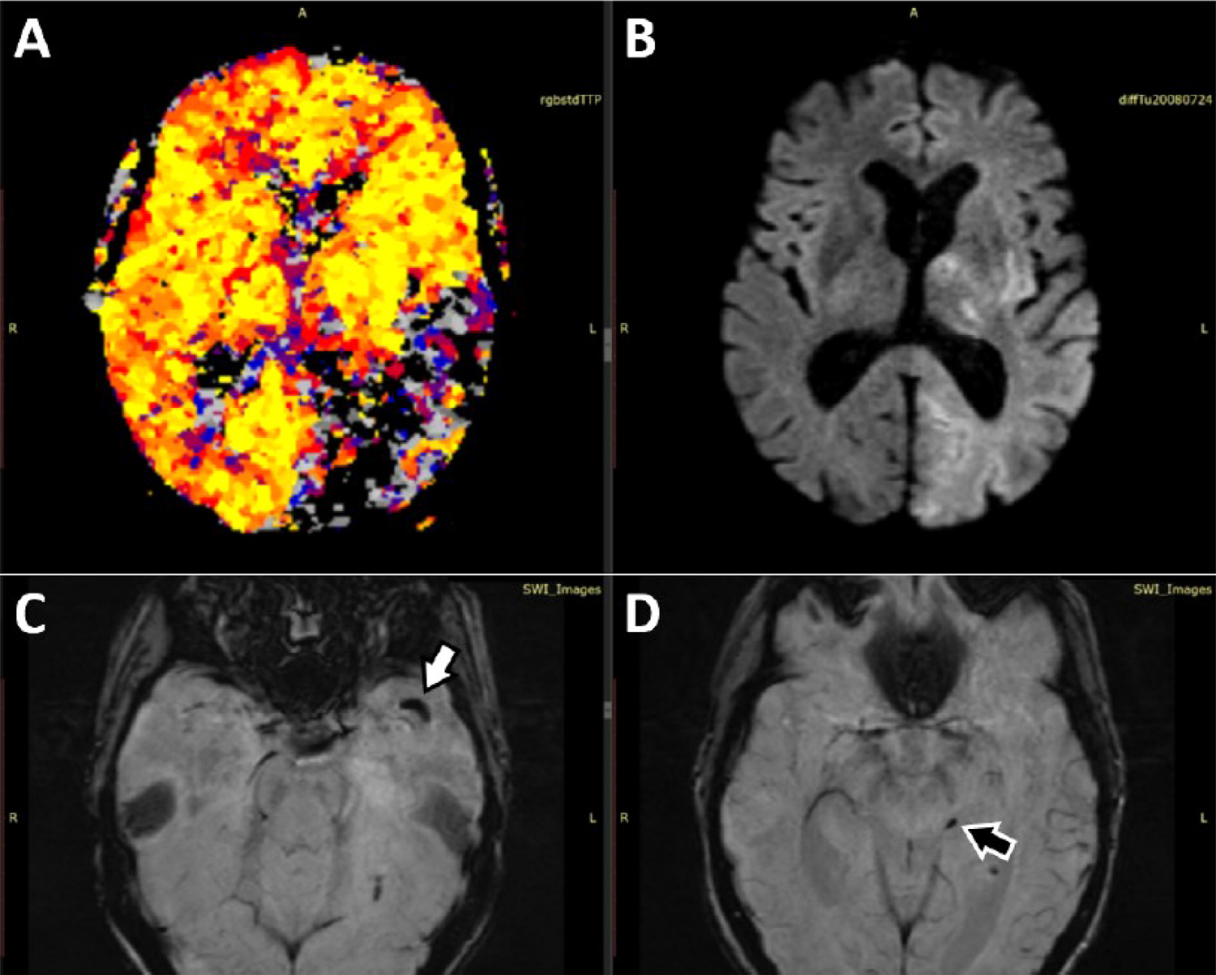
Case 1 - Geometrically matched stdTTP-P-MRI (A) and DWI (B) of case 1 before cTE. After ivTL, migration of the thrombus initially located in the left ICA-C4-segment occurred leading to a perfusion stop (A*: black colored areas*) and adjacent critical perfusion (A: grey colored areas) in the dorsal left MCA- and nearly all parts of the left fPCA-territory. Thrombotic material was depicted in a major M2-branch of the left MCA (C*, white arrow*) and in a P2-branch (D*, black arrow*) of the fPCA in SWI. Due to the severe perfusion restriction an early ischemic injury of the affected tissue became rapidly evident (B*, bright areas in left hemisphere*). cTE, cerebral endovascular thrombectomy; DWI, diffusion weighted; fPCA, fetal posterior cerebral artery; ICA, internal carotid artery; ivTL, intravenous thrombolysis; MCA, middle cerebral artery; P-MRI, perfusion magnetic resonance imaging; stdTTP, standardized time-to-peak parameter; SWI, susceptibility weighted.
